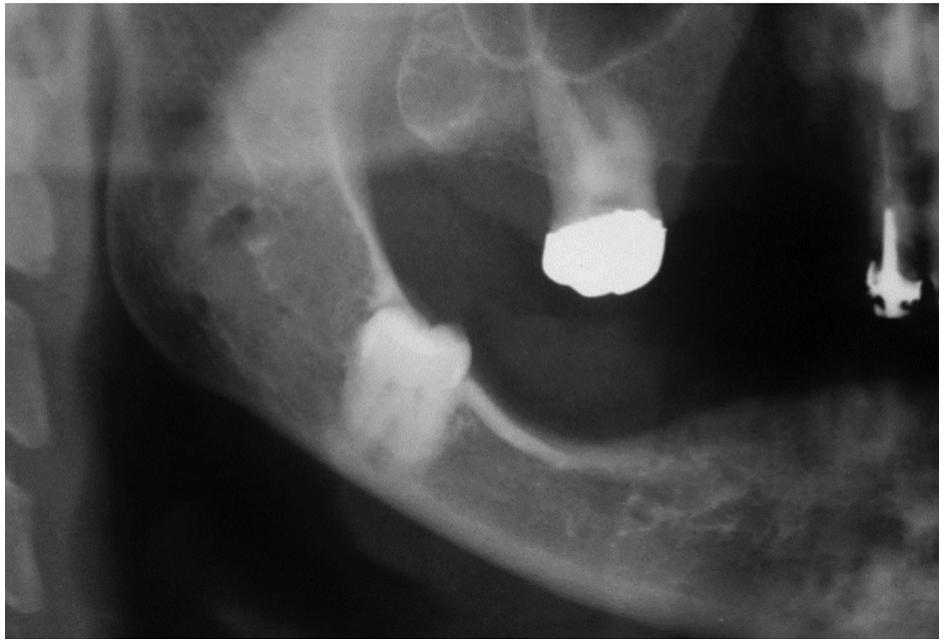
Case 8. Preoperative panoramic radiograph reveals intimate contact of the teeth #48 and inferior alveolar nerve and high-risk of mandibular fracture.
